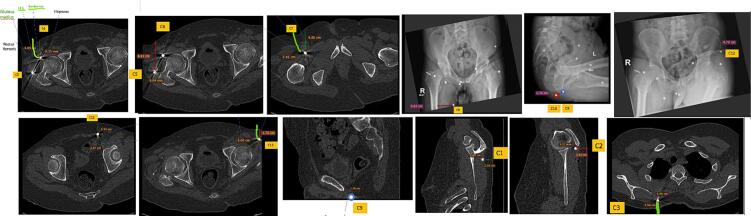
CT scan topographic anatomy of foreign bodies inside the patient.
